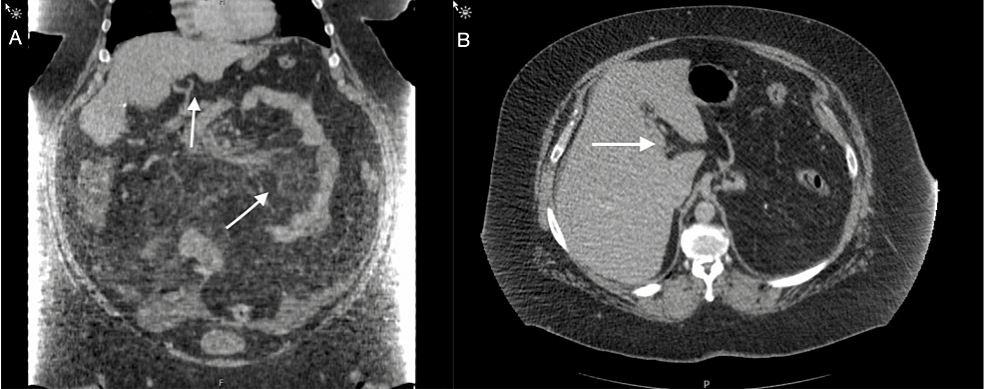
CT showing hazy mesentery, an unremarkable small bowel, and nonvisualization of the portal vein CT: computed tomography (A) Coronal view. (B) Axial view
